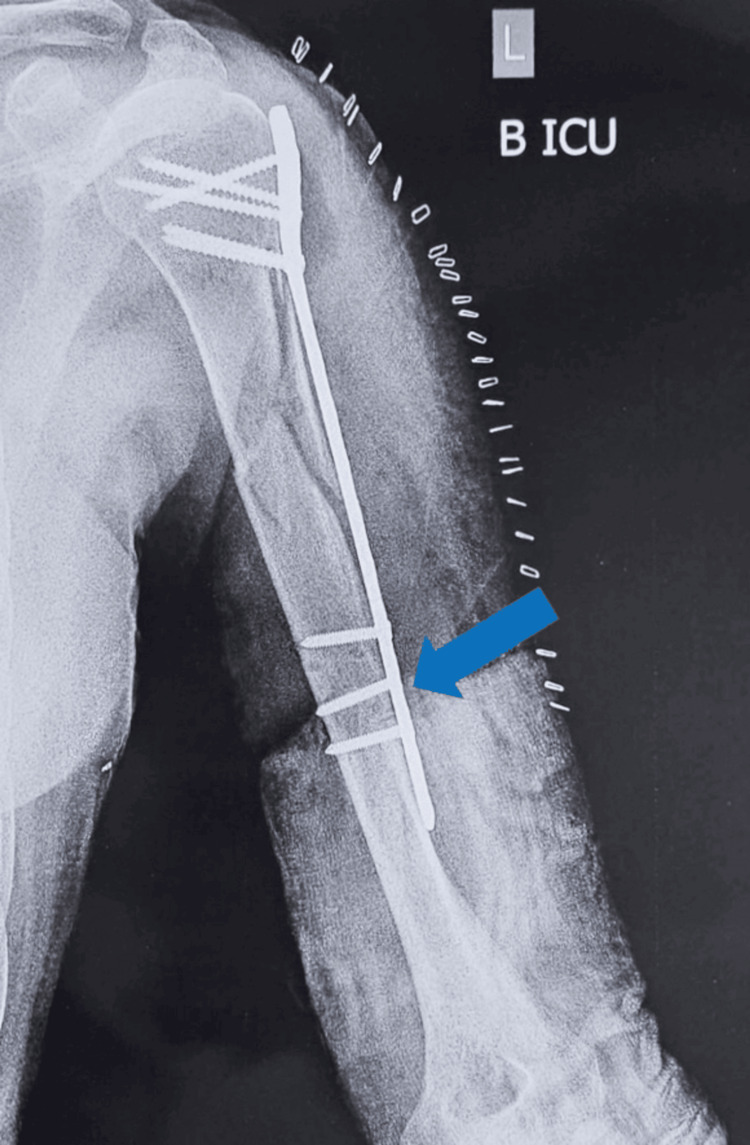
X-ray of left humerus anterior-posterior view. The blue arrow in the figure indicates the site of brachial artery iatrogenic injury.
